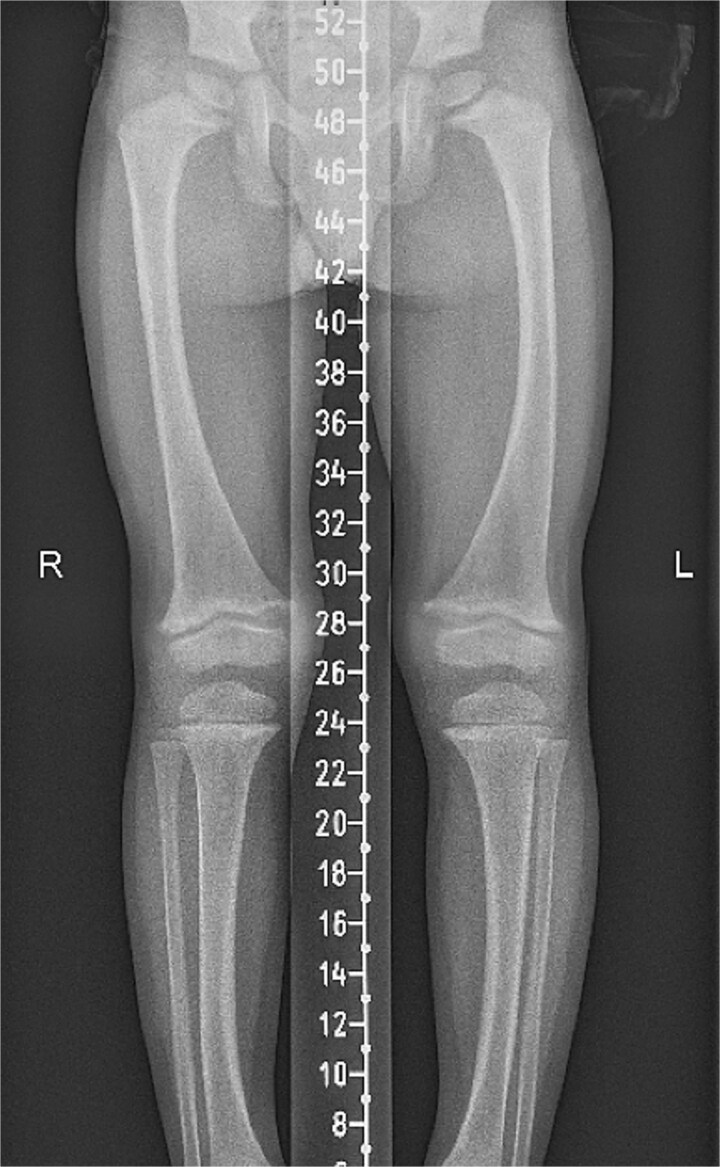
Radiograph demonstrating mild bowing of both femora and tibiae, noting underlying generalized changes of rickets with flaring and cupping of the metaphyses. Borderline bilateral coxa vara.
